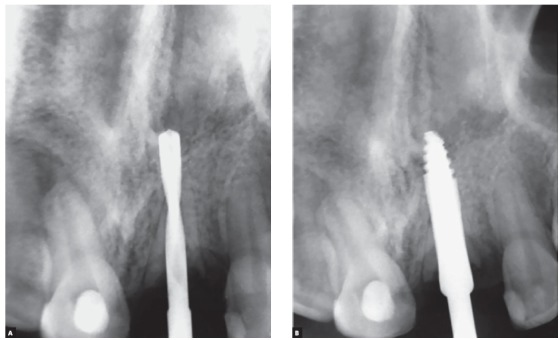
Radiographs of intermediate surgical maneuvers, preparing the place to receive the dental implant, in the patient of the previous figure.
